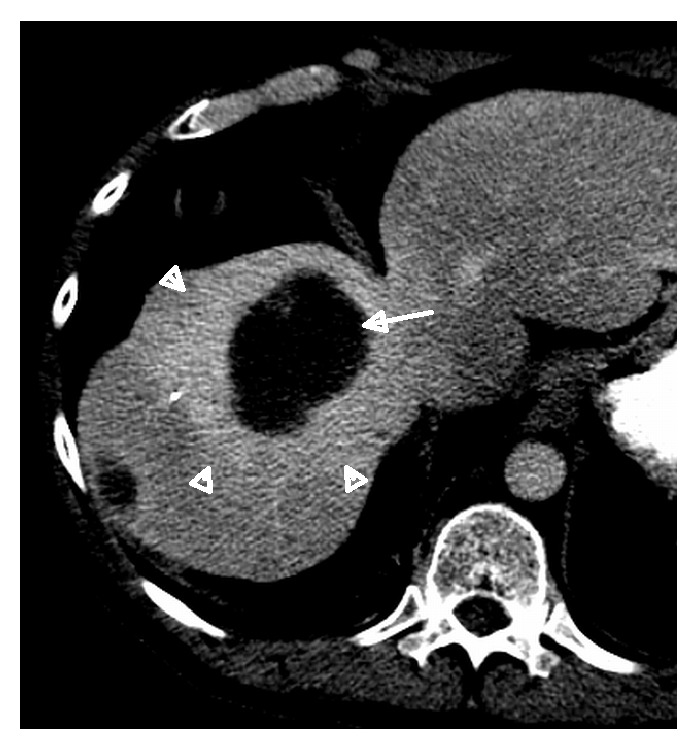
56-year-old male patient with cholangiocarcinoma. Axial CT at delayed phase showing hypodense treated cholangiocarcinoma without residual enhancement (arrow), accompanied by halo of hyperdensity (arrow heads).
